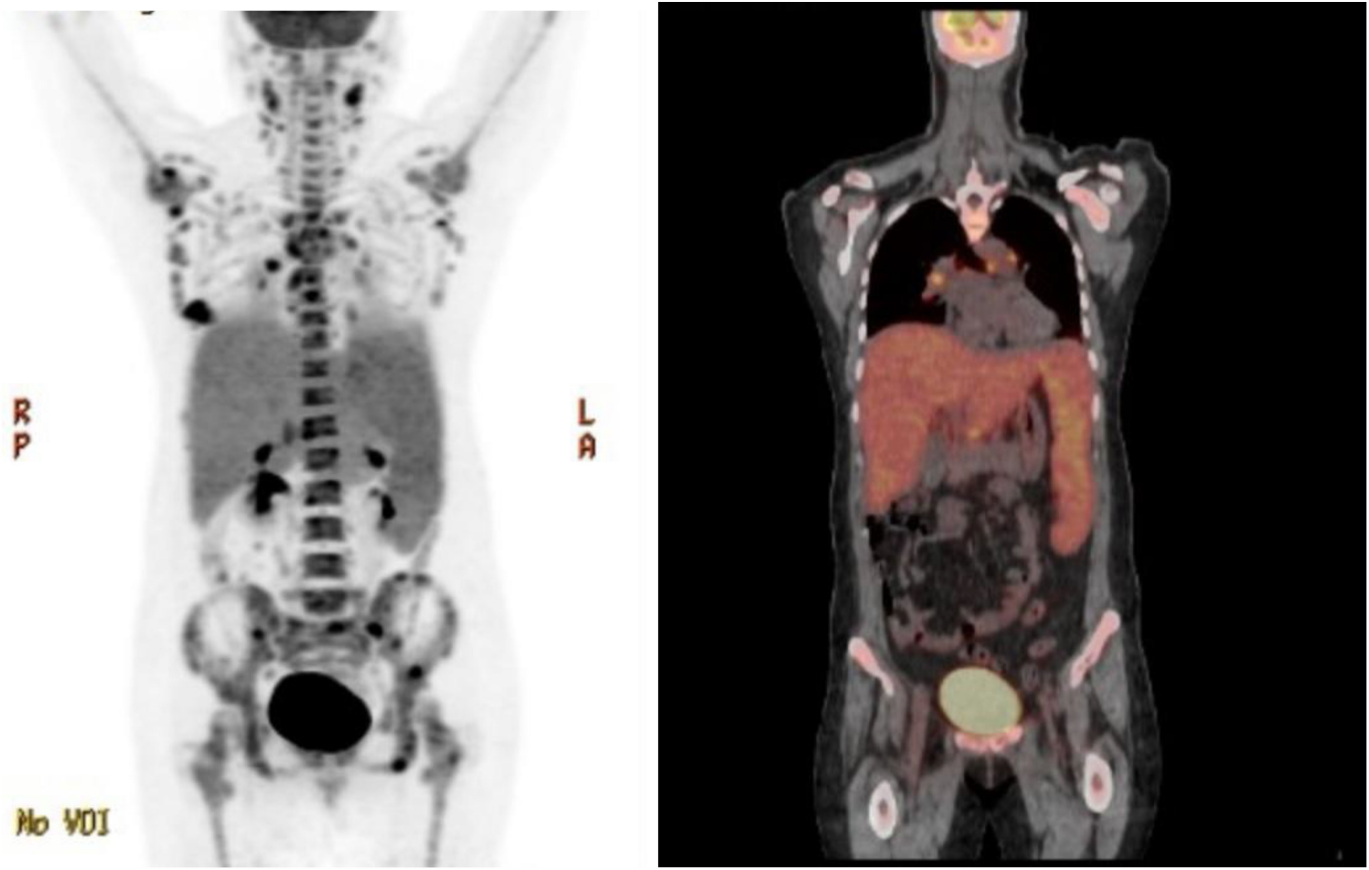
PET-CT highlighting metastatic TNBC with spread to the neck, sternum, thoracic spine, upper abdomen, and pelvis.
